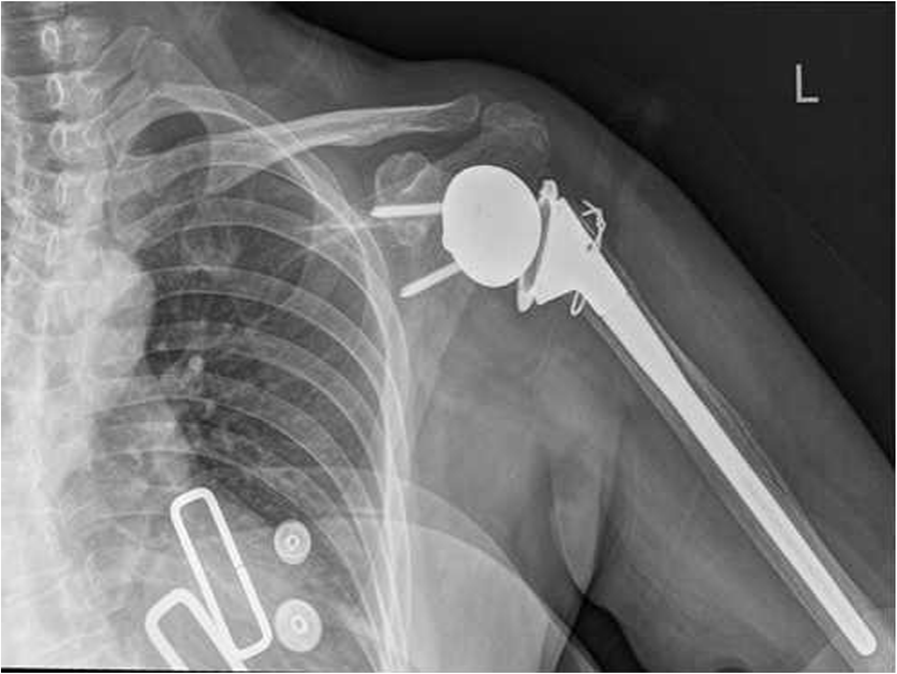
X-ray of the shoulder after the reverse total shoulder arthroplasty
